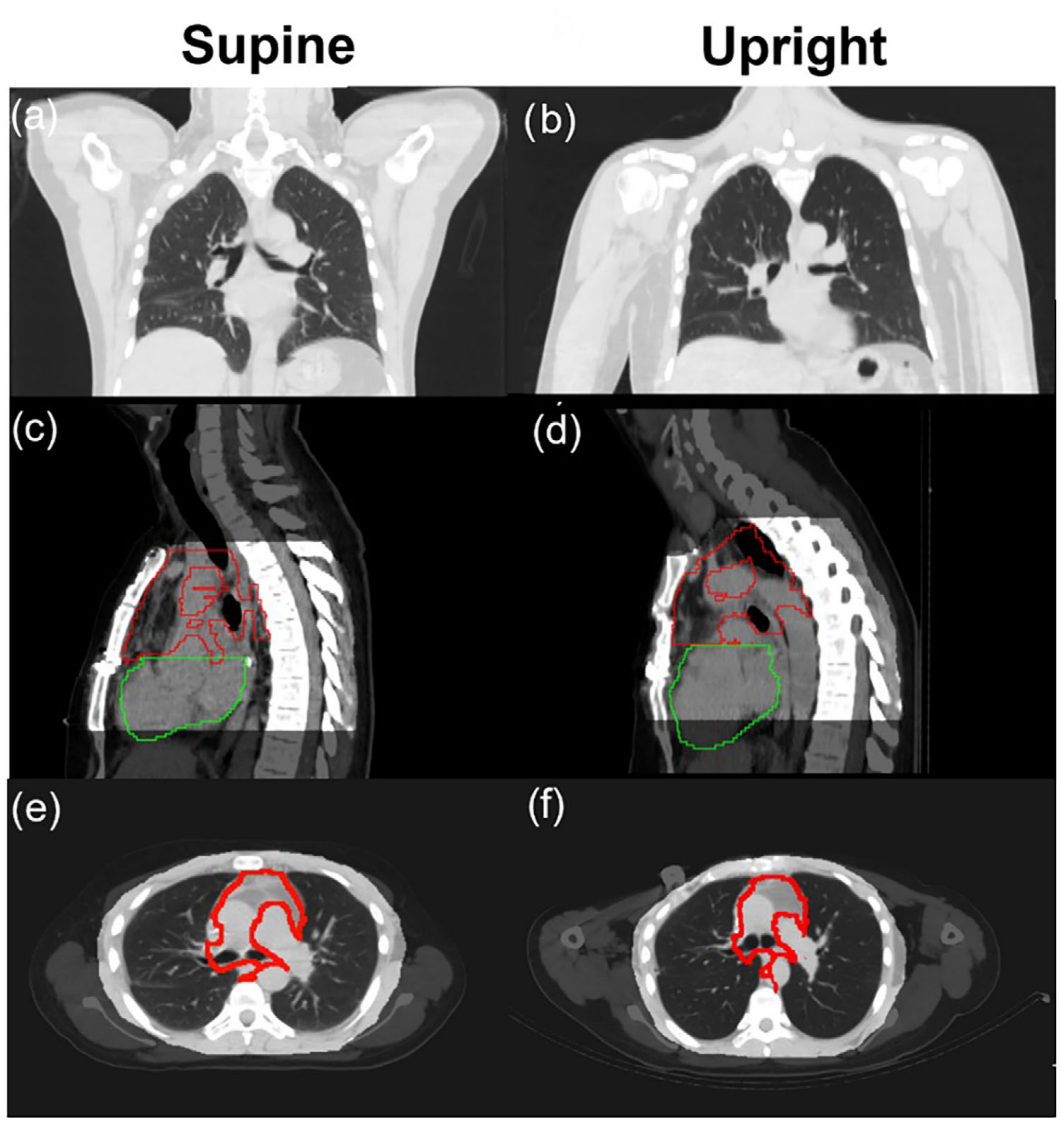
Patient P3 supine (a, c, e) and upright (b, d, f) reference CT in coronal (a, b), sagittal (c, d) and axial (e, f) view. Body position (a, b) and target (red) and heart (green) contour (c, d) differences are highlighted. The ribcage region of interest (ROI) is shown in sagittal (c, d) and axial (e, f) view superimposed to the original CT. The original (e) and propagated (f) target are also shown.
